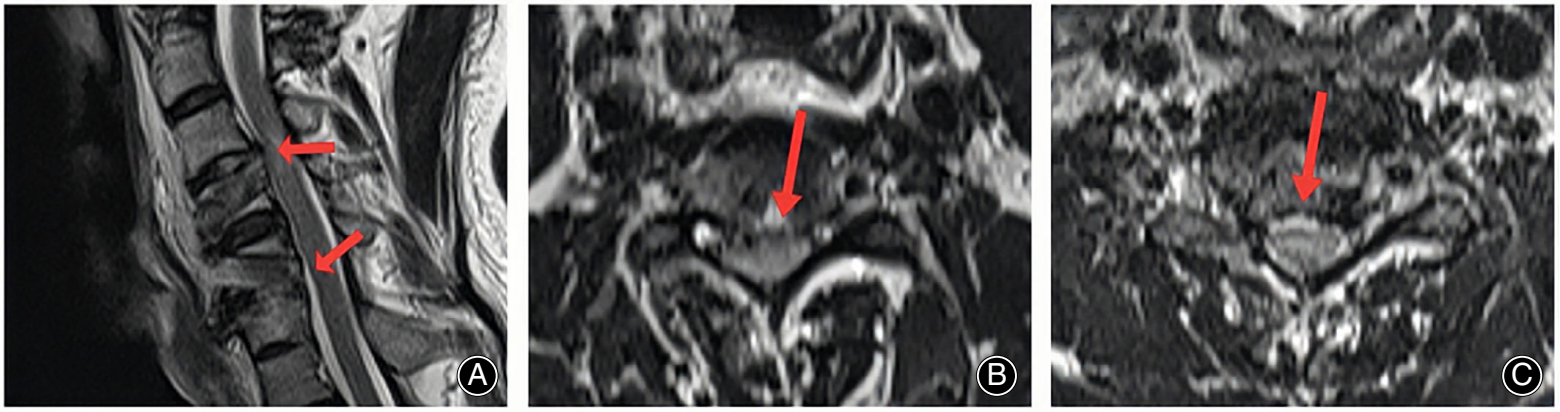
Postoperative T2‐weighted MRI images. The sagittal (A) and transverse images show adequate spinal cord decompression in C3/4 (B) and C5/6 (C).
